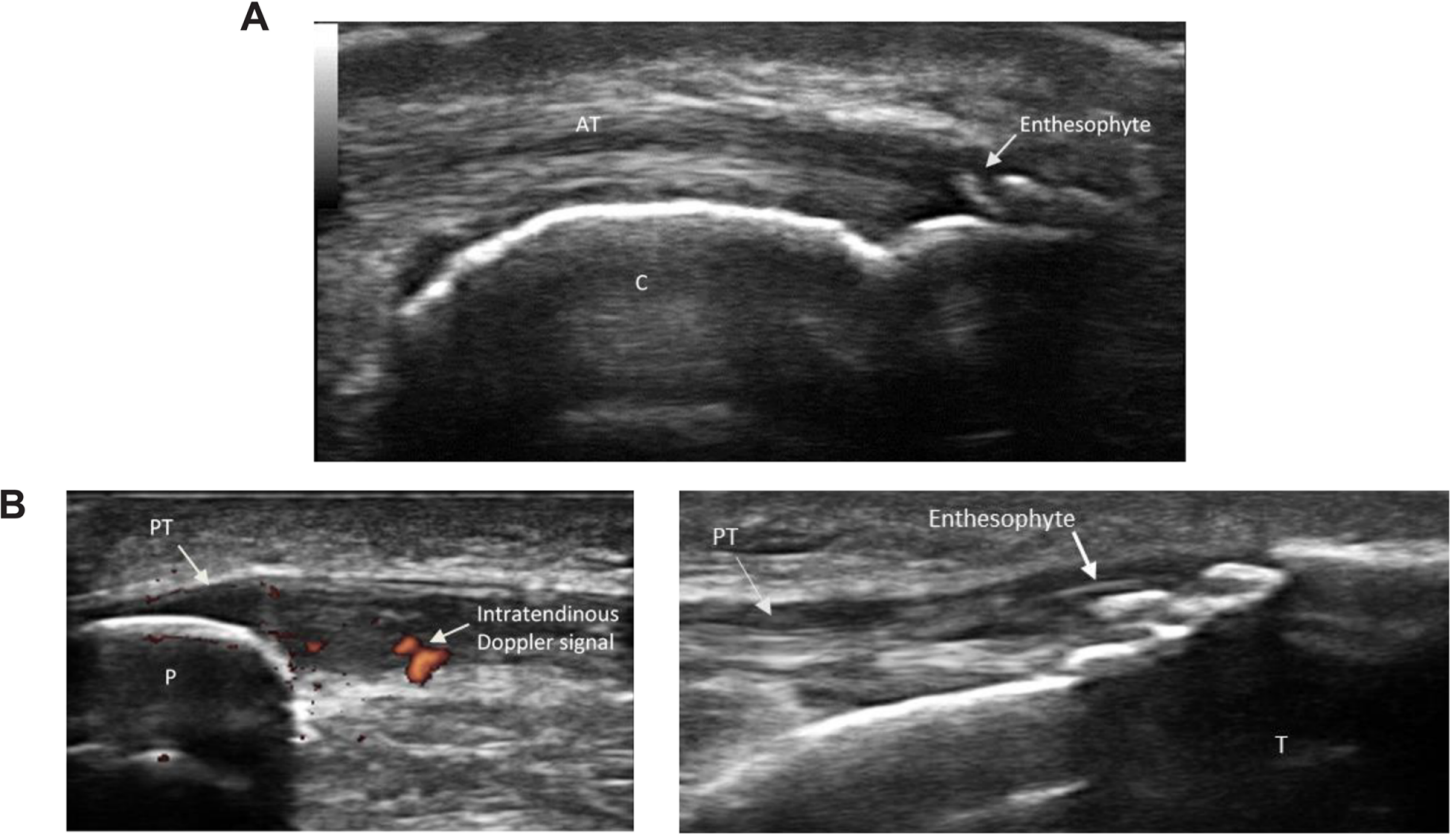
Ultrasound imaging of enthesitis. a Achilles enthesophyte in a patient with spondyloarthritis. AT, Achilles tendon; C, calcaneus. b Patellar enthesitis in a patient with psoriatic arthritis. Left, Doppler with abnormal intratendinous signal; right, enthesophyte. P, patella; PT, patellar tendon/ligament; T, tibia
